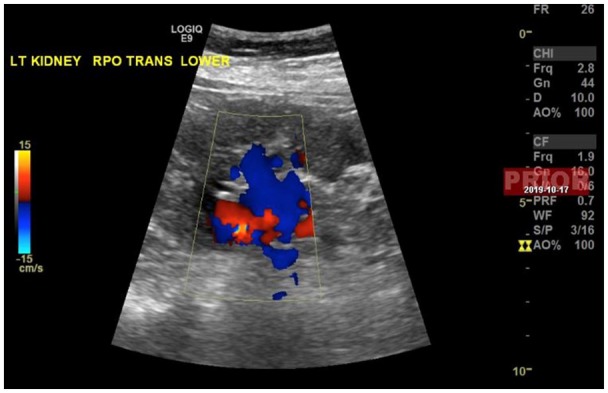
Duplex ultrasound of the left kidney showing patent blood flow to the kidney via the left renal artery.
